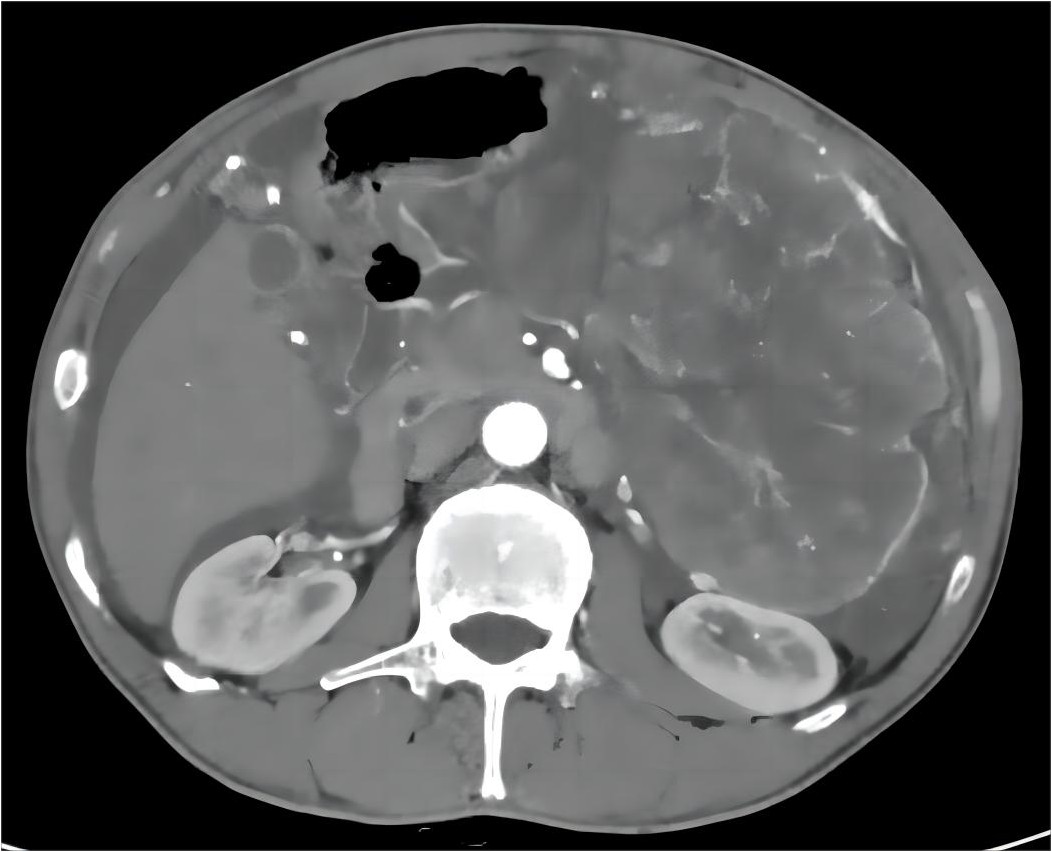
Pre-treatment abdominal CT results with imatinib: A massive tumor on the left side of the abdomen.
